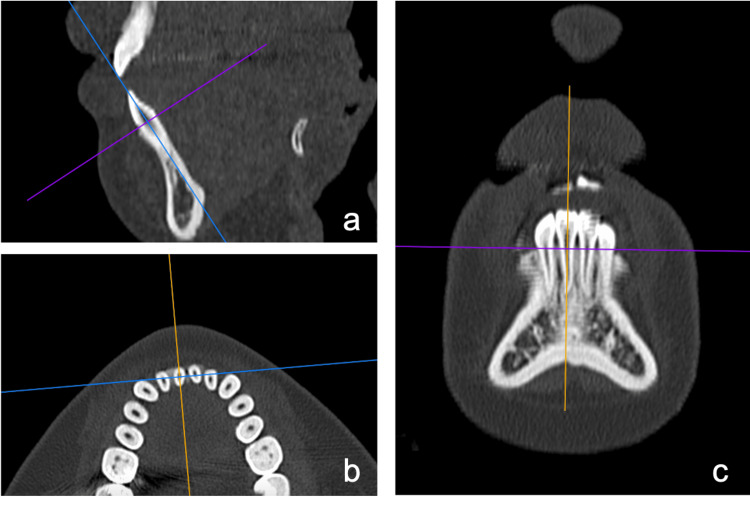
The measurement section of the CT image (a) Sagittal view of lower central incisor; (b) coronal section; (c) frontal section. Image Credits: Yuki iijima
